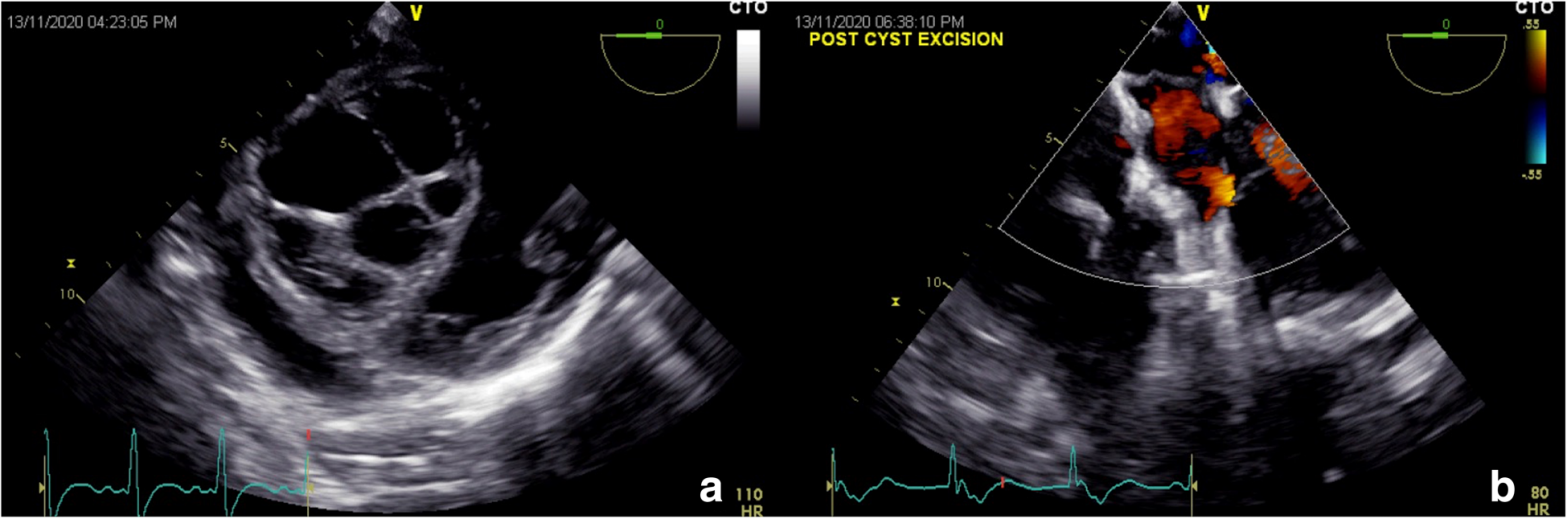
a Intraoperative transesophageal echocardiogram showing multilocular hydatid cyst over the interventricular septum. b Post-excision images showing complete removal of the cyst
